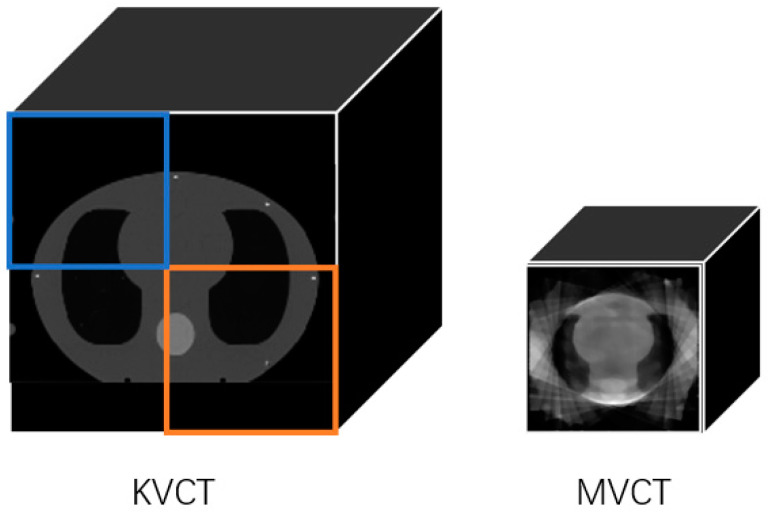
Registration process. Local MVCT is the reference window, while the planning CT is the search area.
